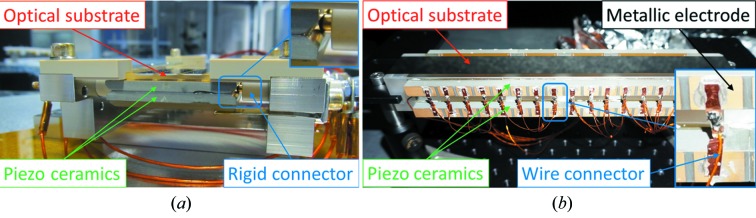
Earlier metrology studies indicated that the rigid electrical connectors [panel (*a*)], which originally supplied high voltage to the piezo ceramics, were resisting the bending of bimorph deformable X-ray mirrors. To correct this issue, TSESO now routinely use flexible wire connectors [panel (*b*)] on more recent bimorphs.
